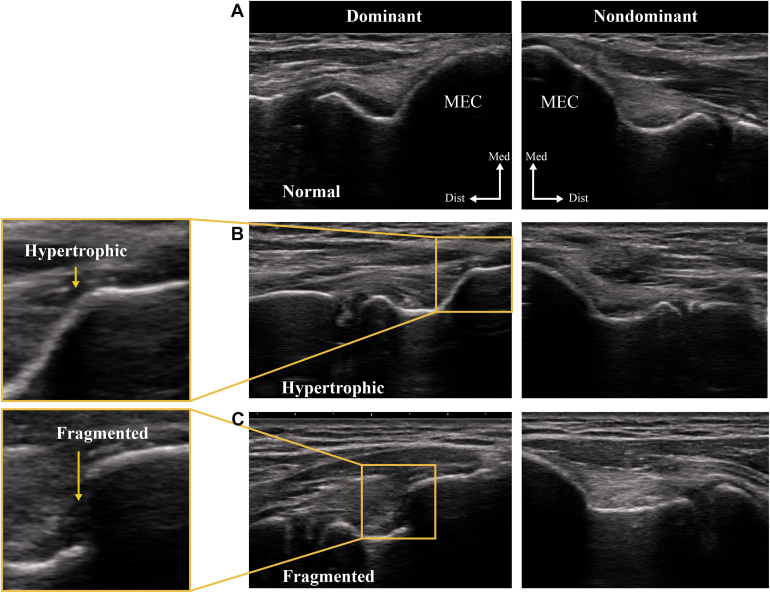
Classification of medial humeral epicondyle morphology. The MEC was evaluated using ultrasonography in the long-axis view. Morphology was classified into 3 categories: (A) normal group, showing an appearance identical to that of the nonthrowing side; (B) fragmented group, showing a clear discontinuity of the cortical margin; and (C) hypertrophic group, showing a distinct cortical protrusion.^30^*MEC*, medial epicondyle; *Dist*, distal; *Med*, medial.
